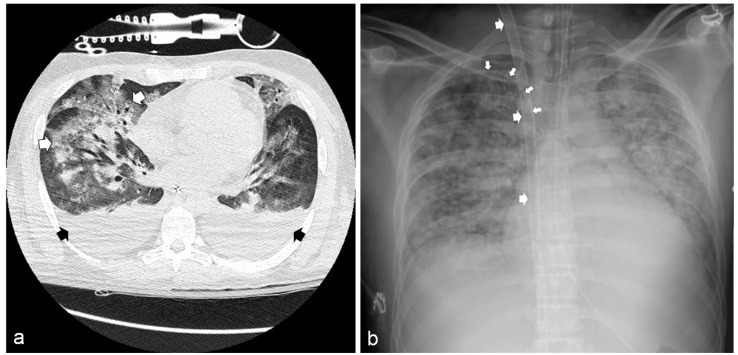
(a) Chest computed tomography showing bilateral pleural effusion (black arrows) and diffuse ground-glass infiltrates (white arrows). (b) Chest radiography showing the bi-caval dual-lumen catheter placed in the right jugular vein (large arrows) and the central venous catheter in the right subclavian vein (small arrows).
